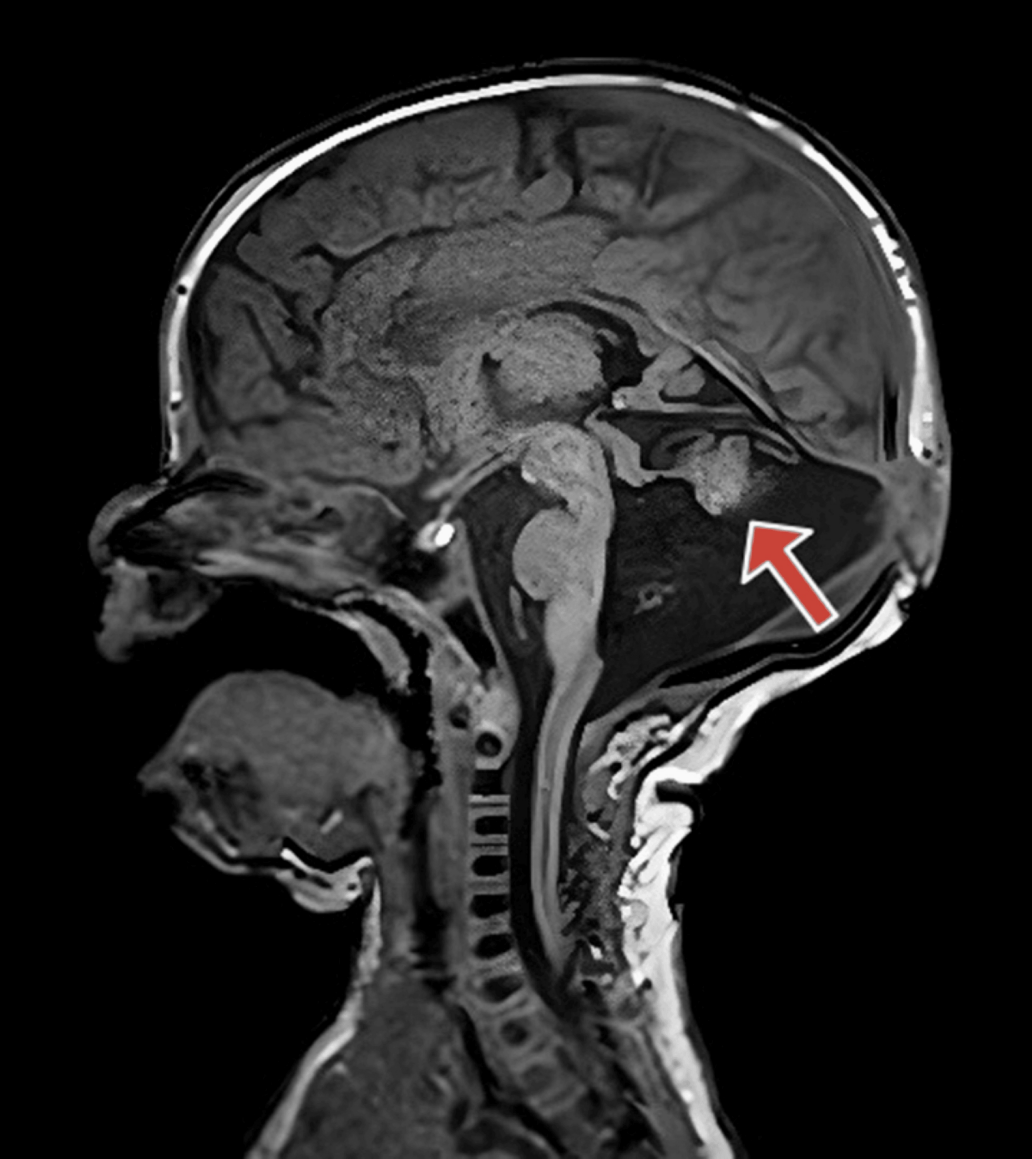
Case 1: MRI brain T1 sagittal image showing the Dandy-Walker feature of cerebellar vermis hypoplasia (arrow) and a small occipital swelling (cephalocele)
